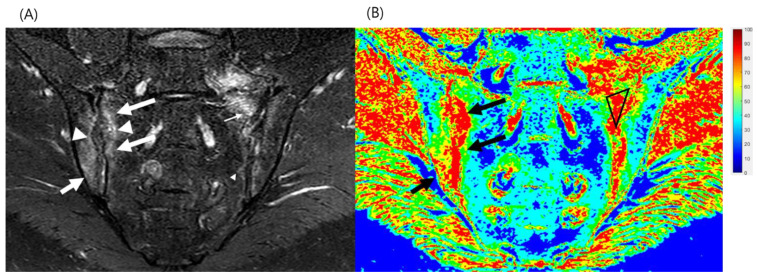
A 56-year-old man diagnosed with ankylosing spondylitis presented with a 2-month history of worsening back pain, morning stiffness, and right-sided Achilles enthesitis. His C-reactive protein level was 74.9 mg/L, while his erythrocyte sedimentation rate was 120 mm/hr. Human leukocyte antigen-B27 was positive. His Ankylosing Spondylitis Disease Activity Score with C-reactive protein was 3.70 and Ankylosing Spondylitis Disease Activity Score with erythrocyte sedimentation rate was 4.17, indicating a very high disease activity state. (A) An mDixon T2 coronal water image showing subchondral bone marrow edema (arrows) and erosions (arrow heads) in both sacroiliac joints. (B) Water fraction map of the sacroiliac joint on mDixon magnetic resonance imaging. The normal marrow regions are blue or green, and the active inflammatory regions (edema) are orange. A polygonal-shaped region of interest over the subchondral bone marrow edema was established to evaluate the water fraction. The mean water fraction value was 0.866.
